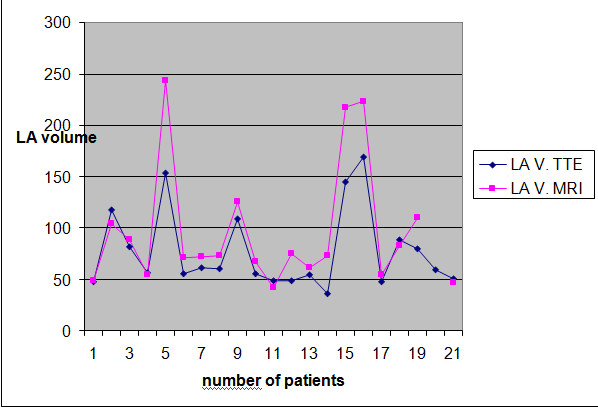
Comparison of left atrial volumes calculated by echocardiography and cardiac magnetic resonance imaging. LA V: Left atrial volume. TTE: Transthoracic echocardiography. MRI: Magnetic resonance imaging.
